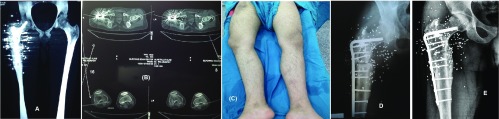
A 22-year old male patient with OGIII-A subtrochanteric femoral fracture due to gunshot injury managed with gamma nail, A) postoperative Scout film, B) postoperative CT scan with 19 degrees external rotation, C) external rotation deformity, D) X-ray after corrective osteotomy, E) 84 months follow up.
